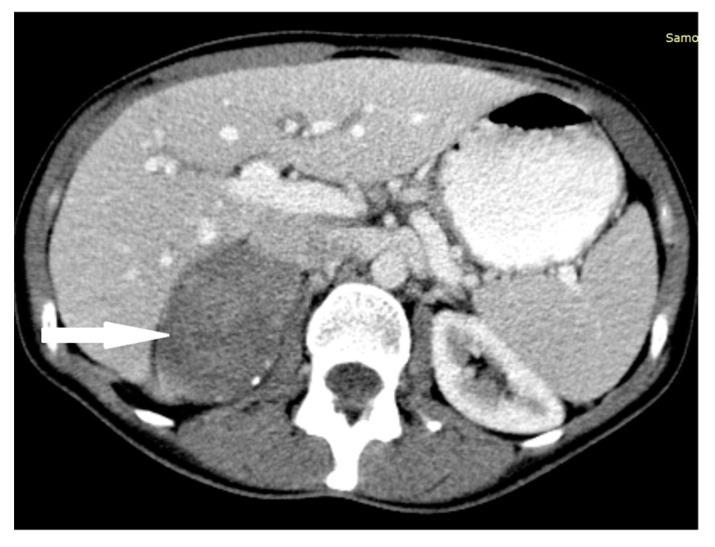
Ganglioneuroma. Abdomen CT, transverse cross-section.
